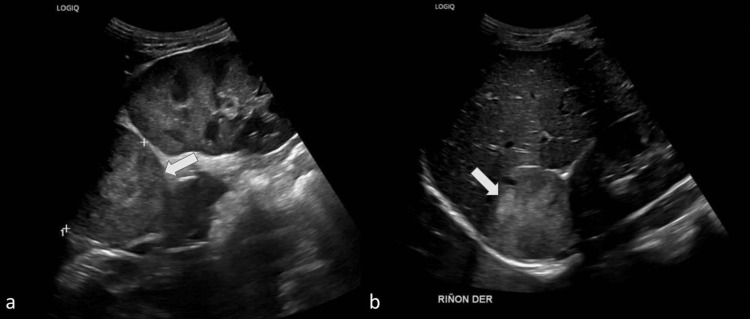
Abdominal ultrasound as part of a secondary hypertension workup demonstrated an incidental right adrenal mass (white arrows)
